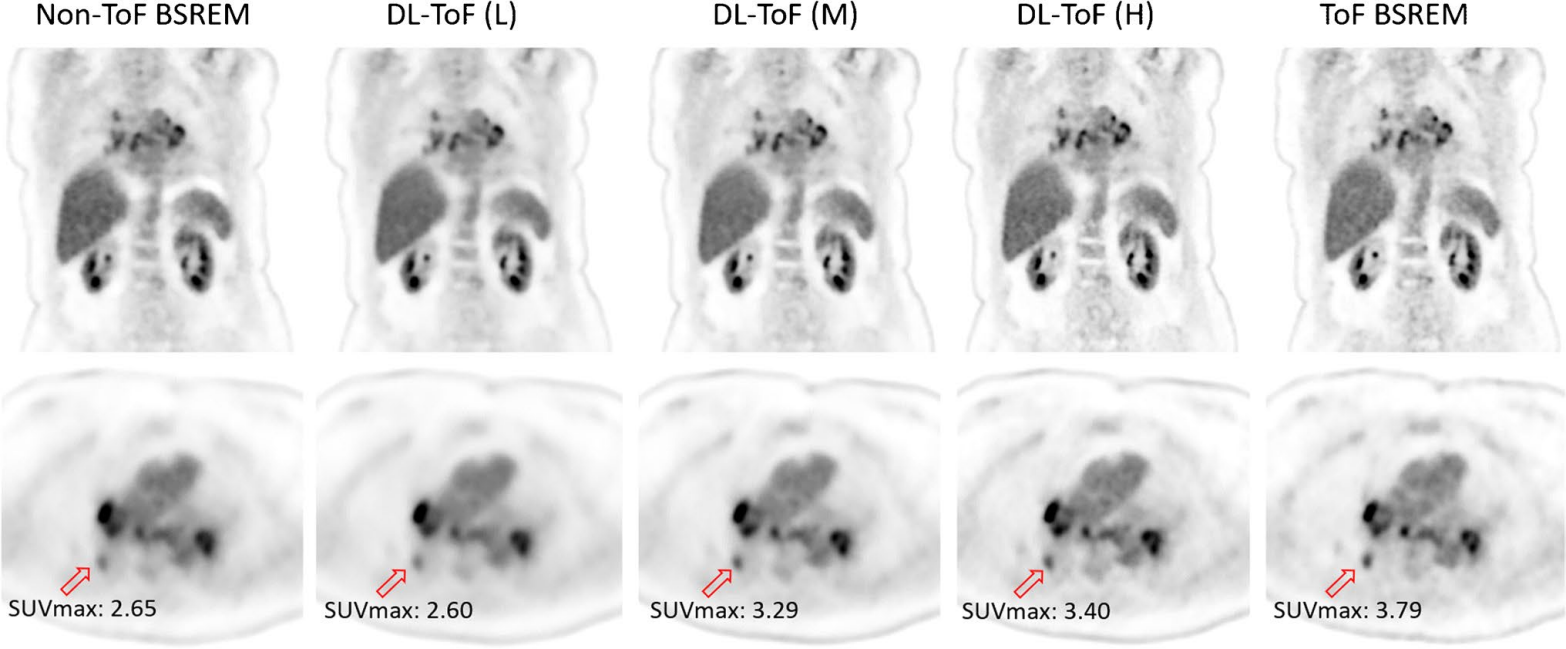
DL-ToF enhancement of a representative test subject with a BMI of 31.6 kg/m^2^ with an injected activity of 514 MBq scanned on GE Discovery MI (5-ring) PET/CT scanner (slice thickness 2.8 mm). Arrows point to an example lesion with SUV_max_ shown (SUV_max_ values of all investigated lesions are summarised in Table 1 and shown in Fig. 3). Display window: 0–5 SUV
